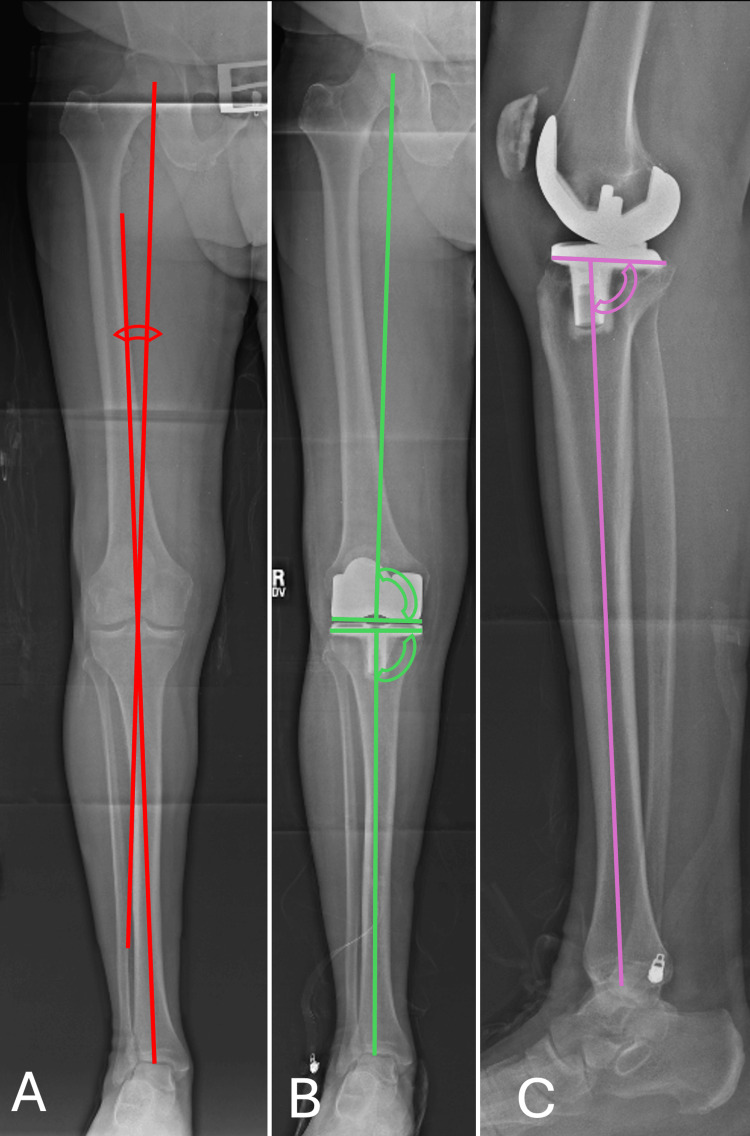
Measurements were recorded via plain radiographs. Panel A: The hip-knee-ankle angle (HKA) is measured in the right knee. Two lines are drawn—one from the center of the femoral head to the center of the intercondylar notch (representing the mechanical axis of the femur) and another from the center of the tibial plafond to the center of the tibial eminences (representing the mechanical axis of the tibia). The angle subtended by these two lines is the HKA. Panel B: The coronal inclination of the tibial and femoral components is measured as an angle between the mechanical axis of the named bone and the joint line of its prosthesis. Panel C: The sagittal inclination of the tibial component is measured using the long-leg lateral radiograph. An angle is measured between the long axis of the tibia and the tibial baseplate. The center of the ankle is used as a distal reference.
